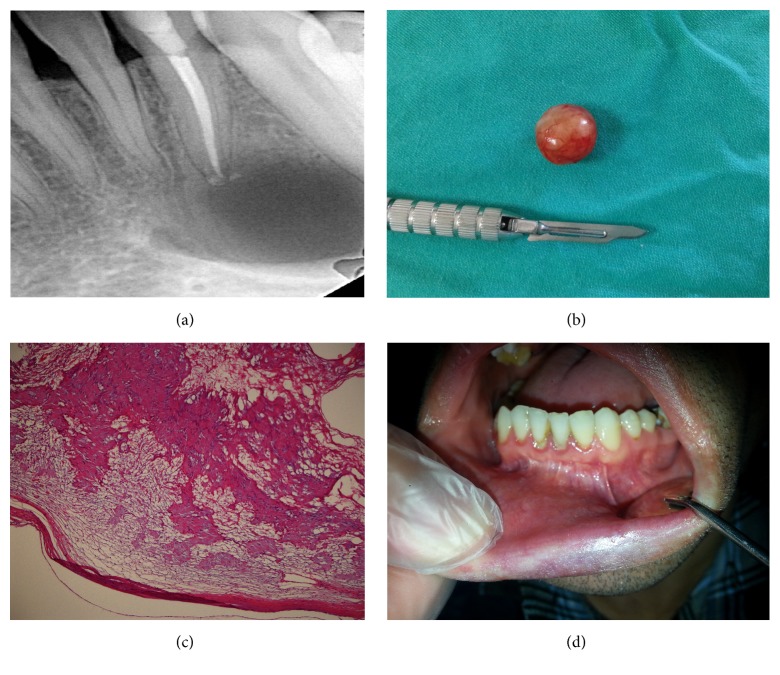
Images of the second case: (a) periapical radiographic image of the mandibular cavity that occurred secondary to schwannoma, (b) enucleated material, (c) fusiform mesenchymal cells forming cellular (Antoni type A) and loose (Antoni type B) areas (40x magnification), and (d) oral cavity at the postoperative 1st year.
